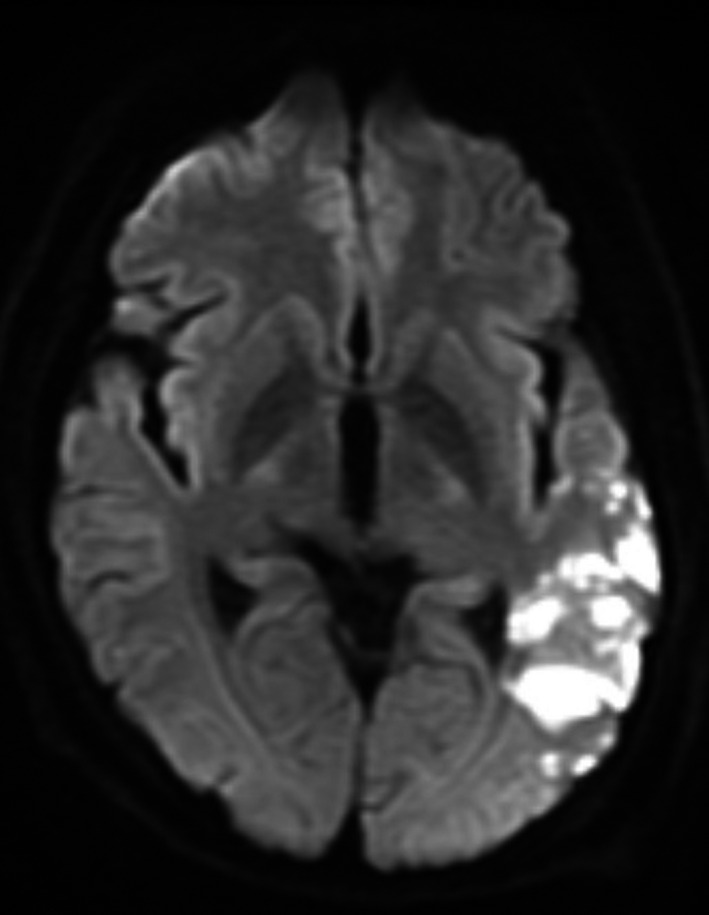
Image diagnosis of the case. Brain MRI showed shower embolization under the cortex of the left temporal lobe and parietal lobe
